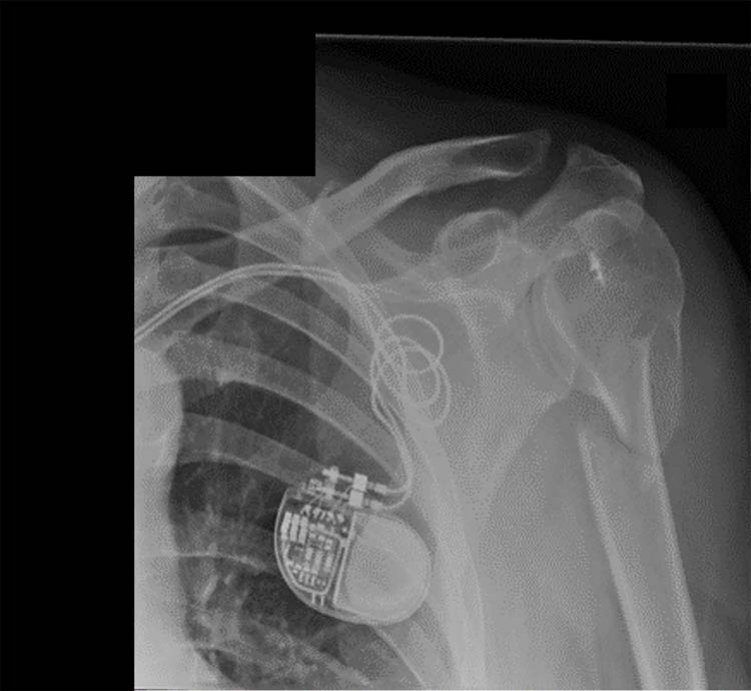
Antero-posterior view X-ray taken 2 weeks post-injury showing acceptable positioning of the fracture.
